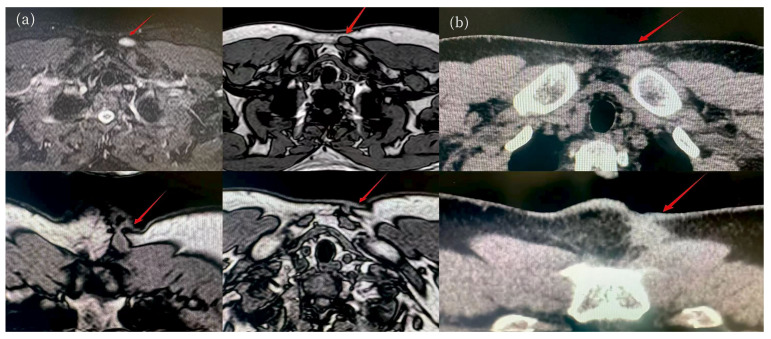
Pilonidal sinus refers to the foreign body reaction elicited by hair embedded within surrounding tissues, most commonly occurring in the sacrococcygeal region, whereas ectopic manifestations on the trunk are rare [1,2,3]. During the acute phase, symptoms typically include redness, swelling, warmth, and pain, with or without purulent drainage. Complications often include difficult-to-heal wounds and the generation of sinus tracts. The clinical manifestations of chronic pilonidal disease vary based on the inflammatory response and skin scarring, with deeper lesions communicating with the skin surface via sinus tracts, allowing for fluid discharge under pressure [4]. We report a case of chest wall pilonidal sinus, highlighting the importance of careful history-taking and considering ectopic pilonidal sinus in the differential diagnosis of refractory chest wall abscesses, so as to avoid misdiagnosis, repeated interventions, and recurrence. Magnetic resonance imaging (MRI) revealed a relatively homogeneous lesion with prolonged T1 and prolonged T2 signal intensities; a cutaneous sinus tract was observed in the anterosuperior aspect of the lesion (a). Computed tomography (CT) demonstrated a well-defined, oval, iso-attenuating nodule measuring 2.0 × 1.0 cm in cross-section, located in the deep subcutaneous fat layer of the anterior chest wall (b), immediately adjacent to the origin of the left sternocleidomastoid muscle and the anterior margin of the subclavius muscle, with preservation of the intervening fascial plane. Pilonidal cysts can occur at virtually any site on the body, yet the sacrococcygeal region and the upper part of the gluteal cleft remain the most common locations. The present case, however, describes an ectopic pilonidal sinus arising from the chest wall that may be related to local repeated friction and minor trauma. Nevertheless, this ectopic entity shares the general clinical features of ordinary sacrococcygeal pilonidal sinus. In the acute phase, it presents with pain, tenderness, and swelling, resembling a superficial abscess elsewhere in the body. Because the lesion is not completely excised, relying solely on local debridement and drainage fails to achieve radical cure, and the disease gradually progresses to the chronic phase. This is characterized by persistent chronic inflammatory changes in the skin and wound nonhealing, with the deep-seated lesion communicating with the skin surface through the sinus tract, from which fluid may exude under pressure. Therefore, whenever a superficial mass recurs or the wound repeatedly fails to heal after excision, particularly if foreign materials such as hairs are discovered in the wound, the possibility of pilonidal sinus must be considered, regardless of the anatomical location. For diagnostic imaging, CT and MRI are distinctly superior to color Doppler ultrasonography, as they are more effective in delineating the underlying sinus tract. Once the diagnosis of pilonidal sinus is established, prompt treatment is warranted; however, complete surgical excision remains the only definitive curative approach. In our case, the patient underwent total excision of the lesion, and the postoperative course was uneventful. The wound healed well, and sutures were successfully removed on the seventh postoperative day. The patient was followed up a month post-surgery, with satisfactory wound healing, and there has been no recurrence to date.
